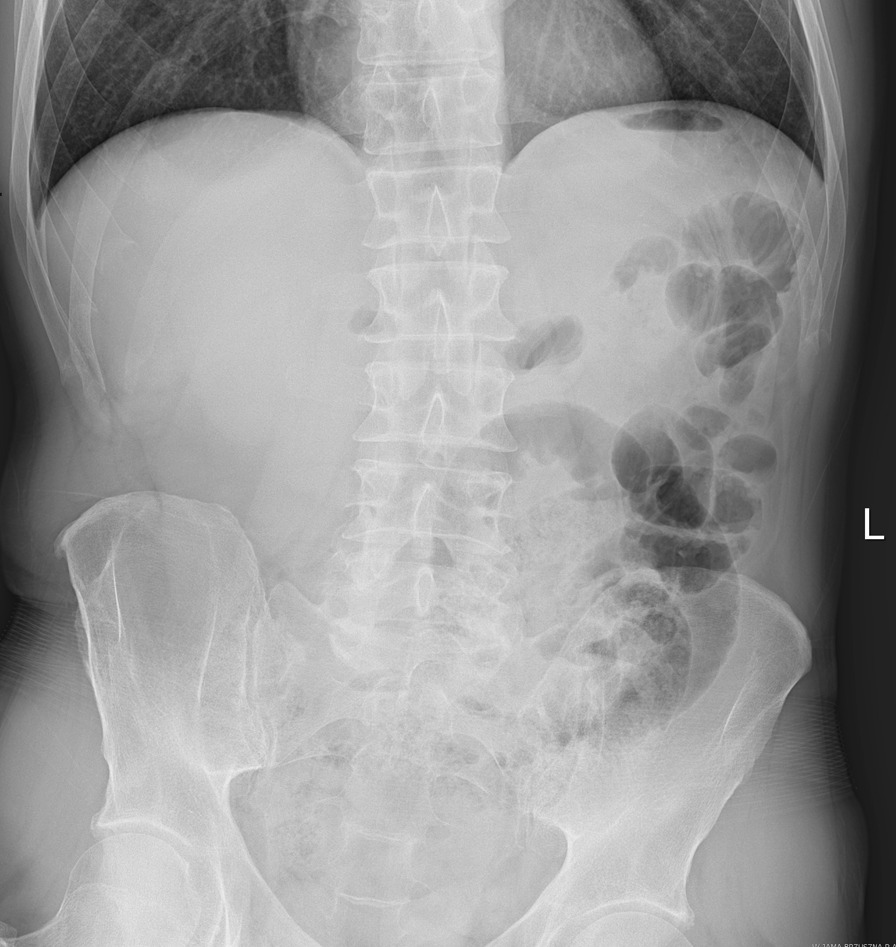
Abdominal X-ray, dilated loop of the small intestine in the lower abdomen
